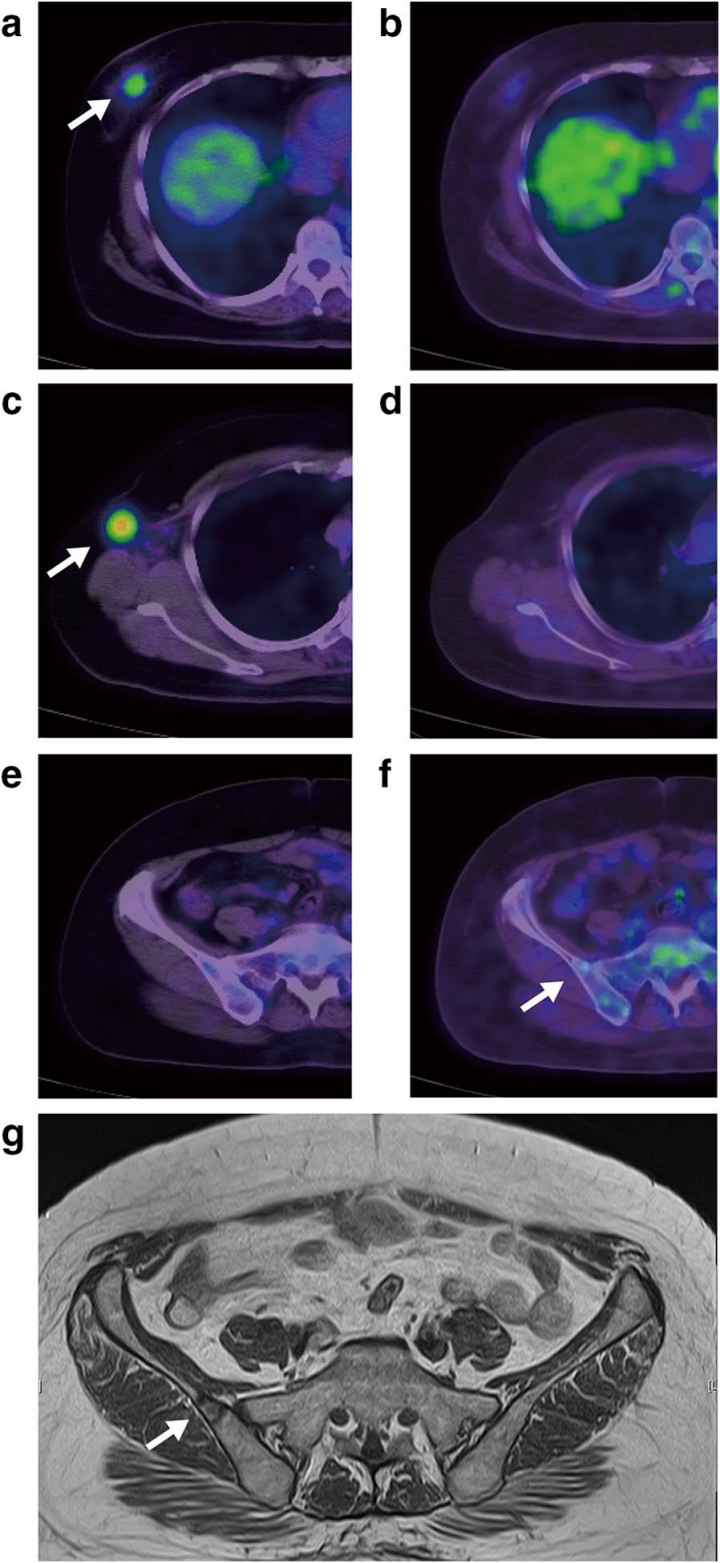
A 56-year-old woman with detected bone metastasis using FDG-PET/CT after NAC. a, b The abnormal FDG uptake of the primary lesion was 3.7 (a) before NAC and 1.4 (b) after NAC. c, d The abnormal FDG uptake of the axial lymph node was 6.1 (c) and disappeared after NAC. e, f Abnormal FDG uptake of the right ilium was not detected (e) before NAC and was 1.8 (f) after NAC. g Bone metastasis was varied in right ilium on MRI
